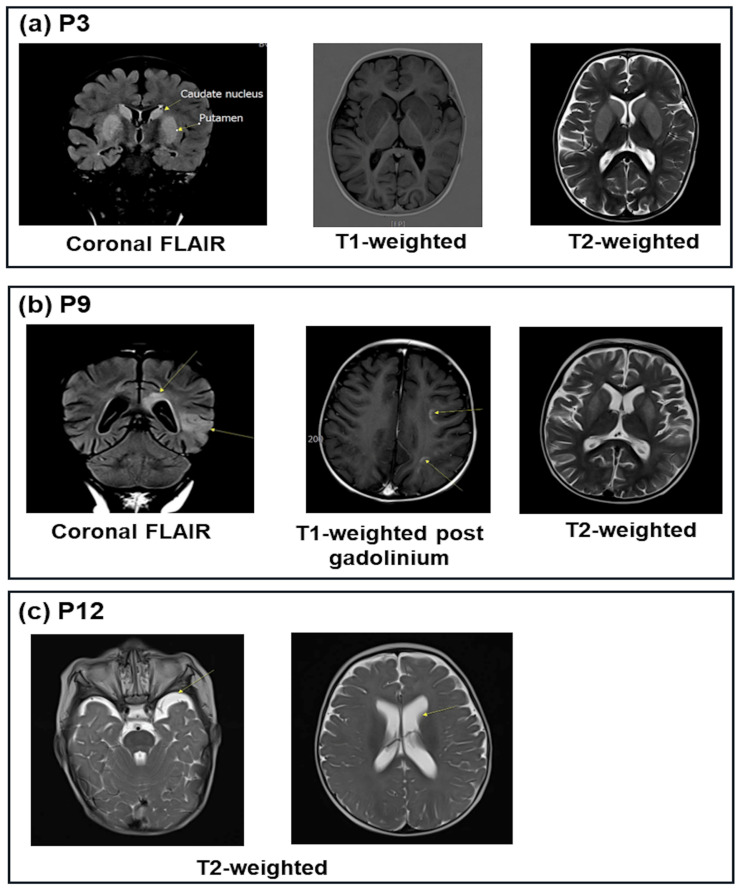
Magnetic resonance imaging (MRI) of three Vietnamese cases with mitochondrial 3-hydroxy-3-methylglutaryl-CoA synthase deficiency (HMGCS2D). (a) P3: coronal FLAIR, axial T1-weighted, and axial T2-weighted images showed symmetric hyperintensities involving both the head of caudate nuclei and putamina. (b) P9: coronal FLAIR image showed bilateral hyperintensities of periventricular deep white matter and left parietal cortex; axial T1-weighted post-gadolinium image showed enhancement of the subcortical area in the left frontal and parietal lobe; axial T2-weighted image showed bilateral symmetric hyperintensities involving putamen. (c) P12: axial T2-weighted MR image showing bilateral ventricular dilatation and the widening of temporal subarachnoid space predominant on the left side.
